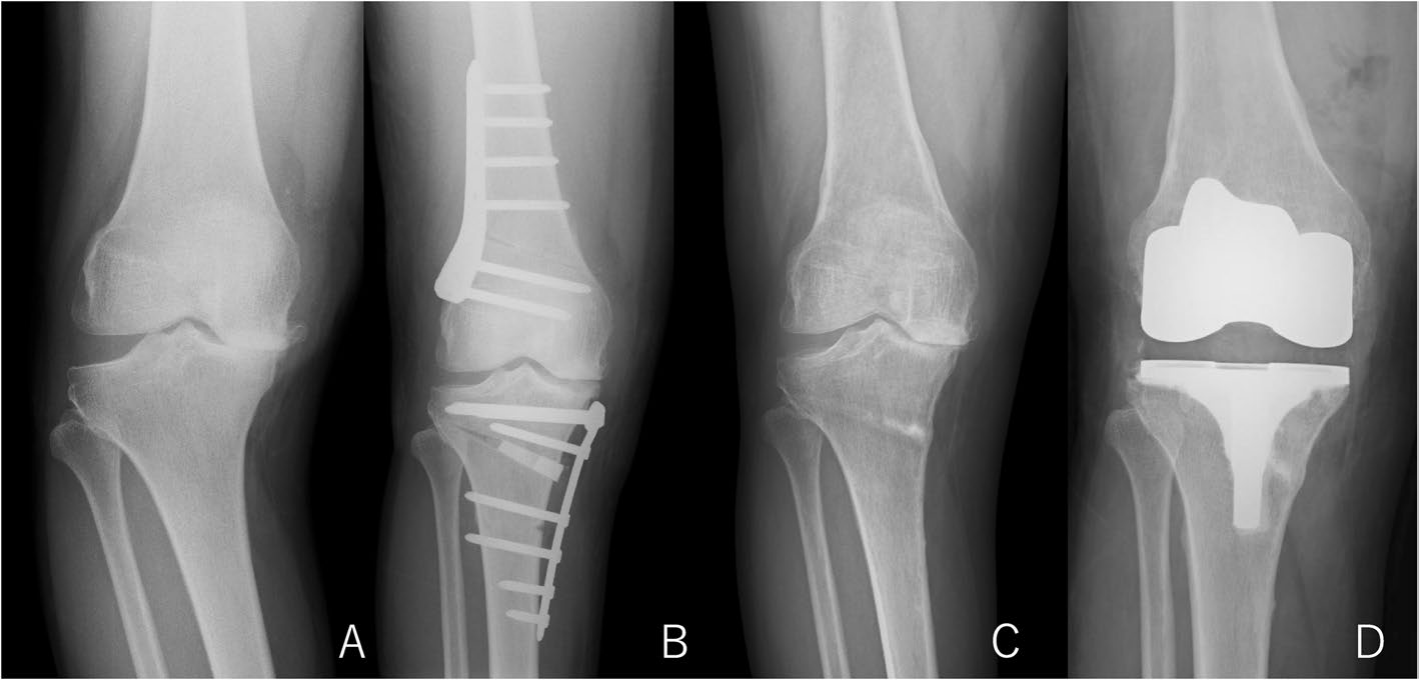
Serial radiographs of the right knee converted to TKA following DLO, A: A weight-bearing anteroposterior radiograph preoperative JLCA is large (9.7°). B: An anteroposterior radiograph following DLO. C: A weight-bearing anteroposterior radiograph 2 years after DLO. Varus alignment was found to be associated with recurrence of large JLCA. D: An anteroposterior radiograph following TKA conversion, JLCA: joint line convergence angle, DLO: double level osteotomy, TKA: total knee arthroplasty
